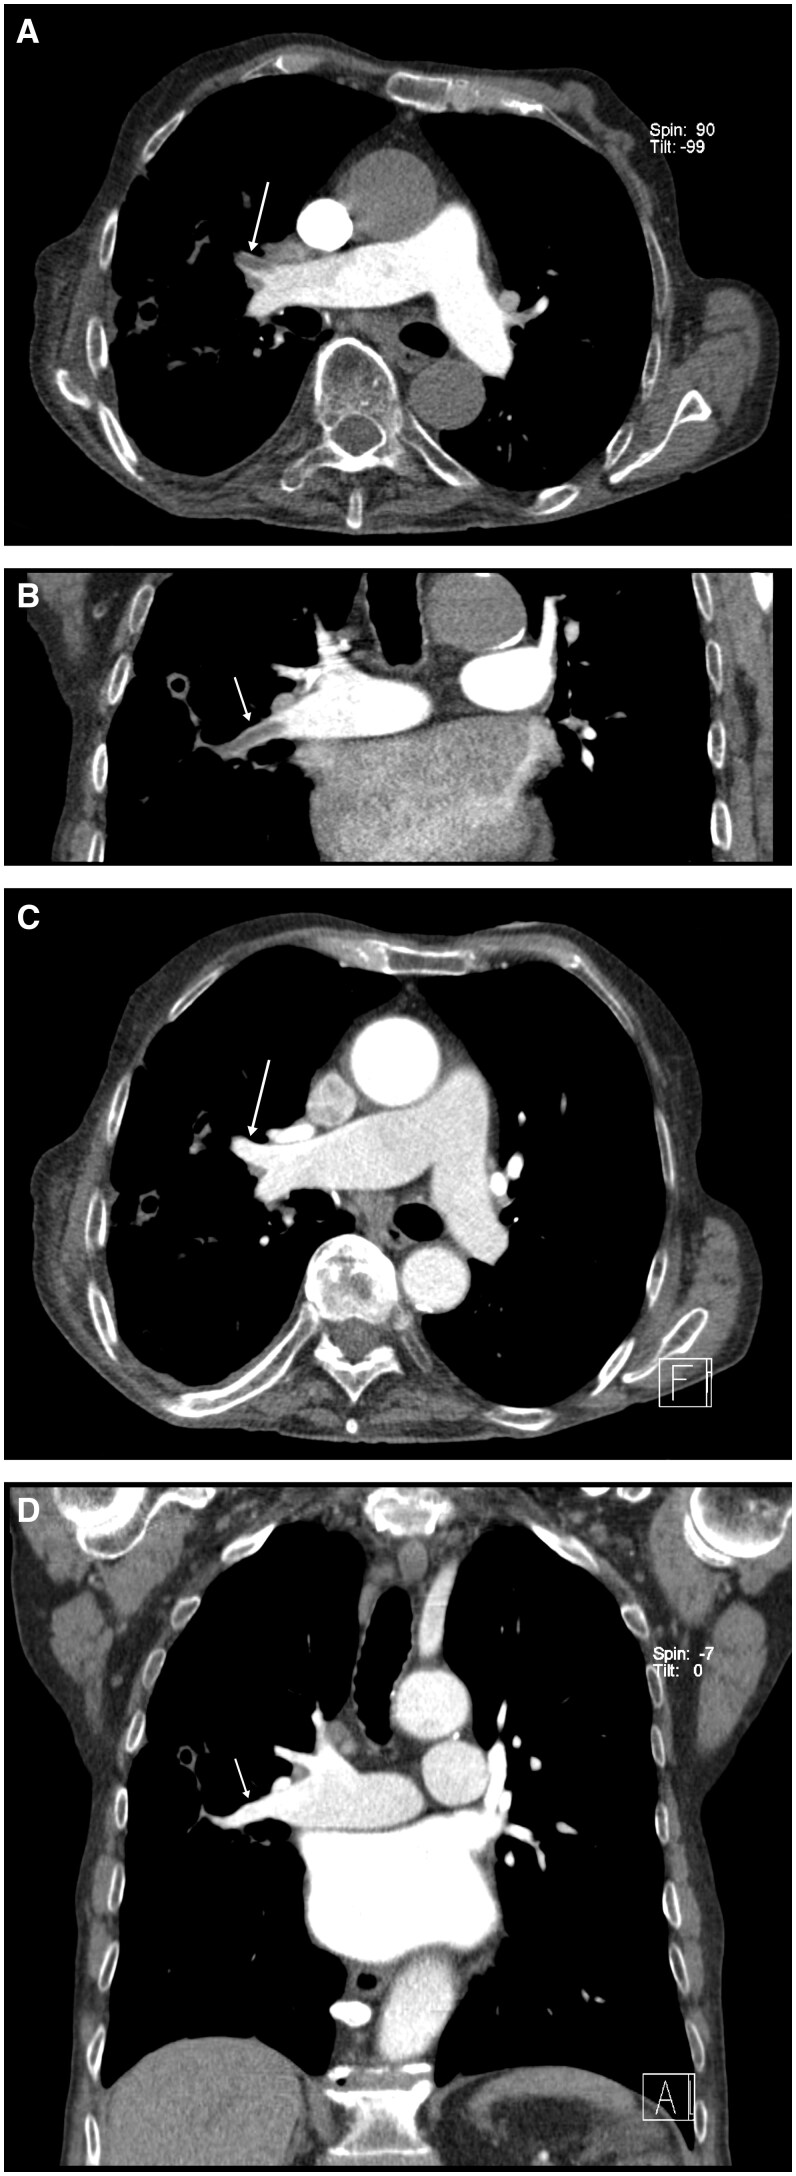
Contrast-enhanced CT. (A) Axial oblique plane: pulmonary phase with filling defects of middle lobe artery (arrow) consistent with pulmonary embolism (seemingly incomplete enhancement of the left posterior segmental artery due to partial volume effect). (B) Same situation in coronal oblique plane (arrow). (C) Axial oblique plane: aortographic phase with disappearance of filling defect (arrow). (D) Same in coronal oblique plane (arrow).
